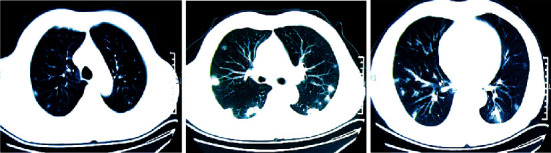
Chest CT scans of a COVID-19 pneumonia patient on day 5 of the onset of symptoms.
